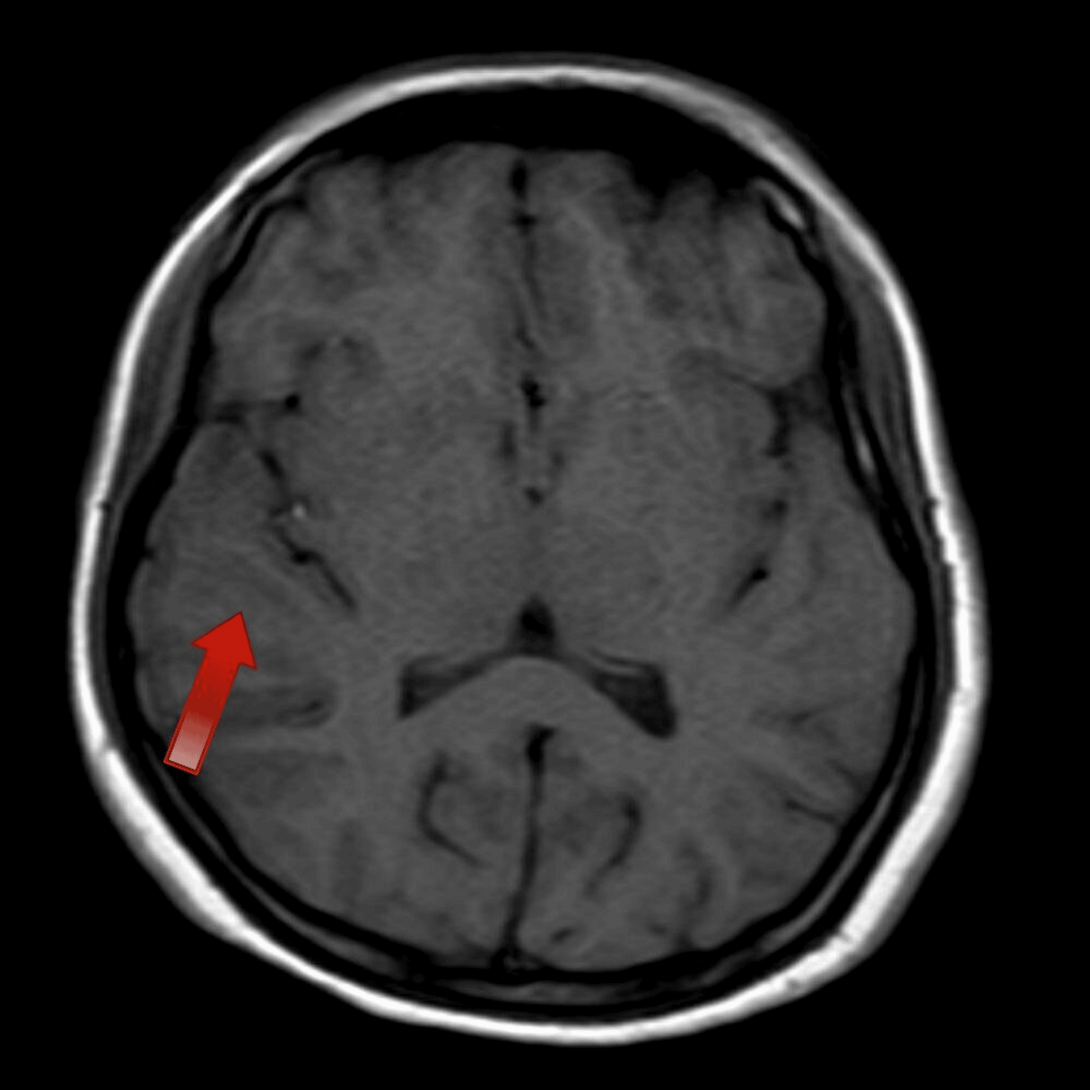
MRI image-T1 showing hypointense. This MRI image obtained using the T1-weighted sequence displays hypointense regions (areas appearing darker) within the brain.
